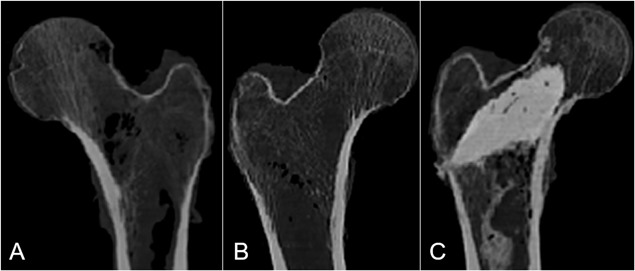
Pre‐ and post‐treatment CT images of osteopenic paired femurs. Control (A), Treated before debridement and AGN1 injection (B), and after AGN1 injection (C).
